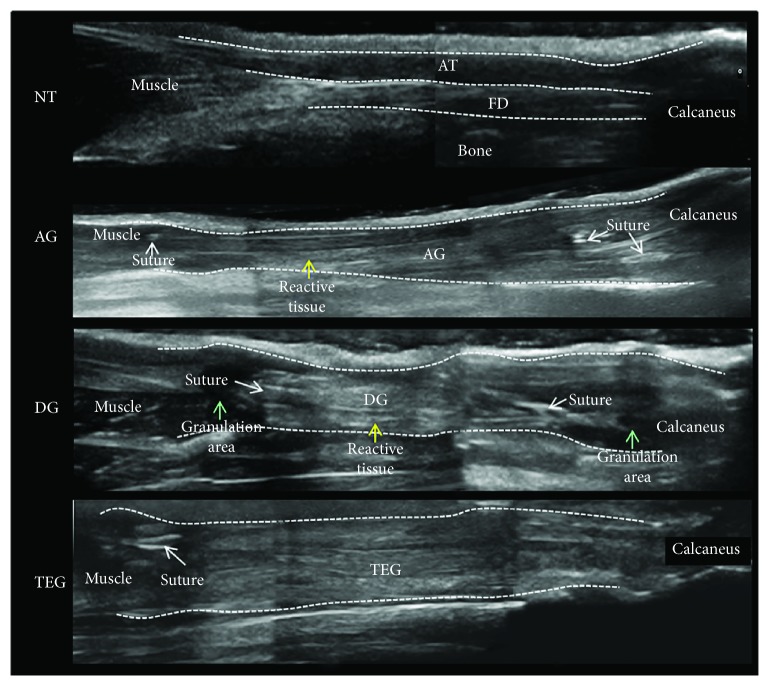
High-frequency ultrasound images of the longitudinal plane of rabbit Achilles tendon complex (AT) and flexor digitorum superficialis (FD) at proximal, mid-, and distal section of the native tendon (NT) and treated groups (AG, DG, and TEG).
